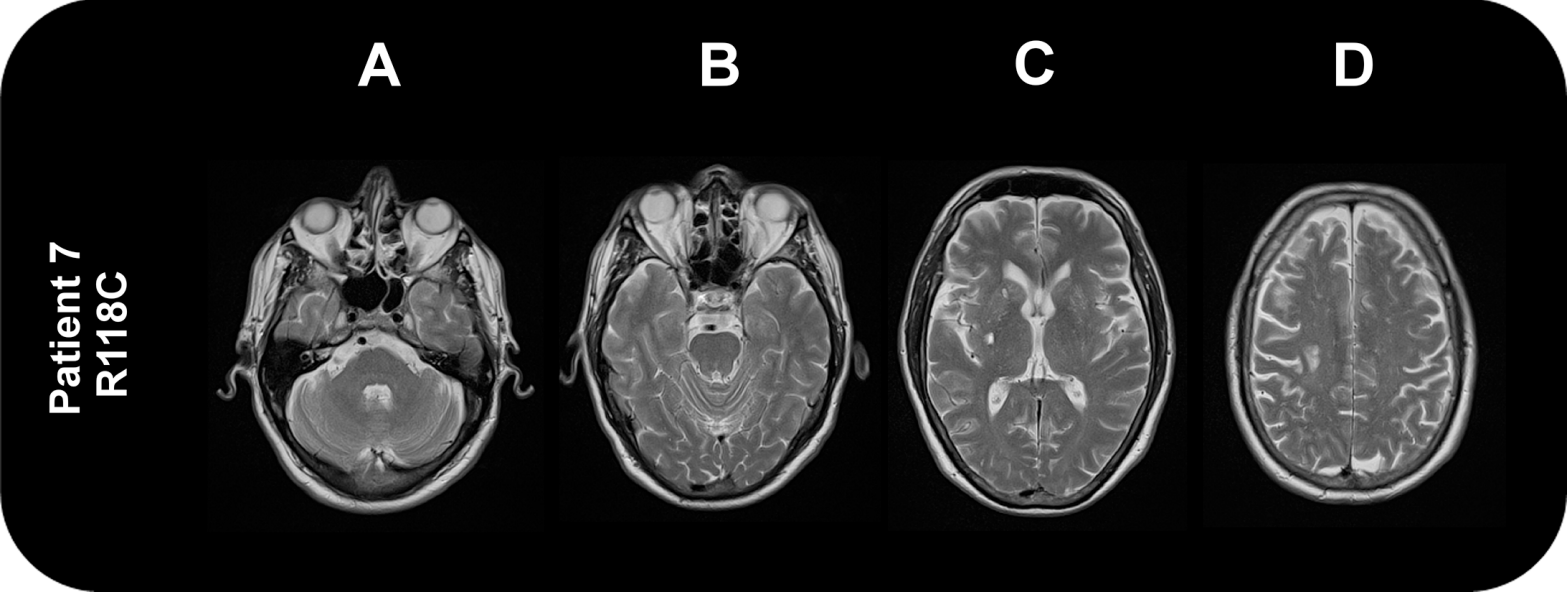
Brain MRI (T2-Weighted) of the patient carrying the *GLA* mutation R118C. Two lacunes are present in the right caudate nucleus and external capsule (C). An area of white matter hyperintensity is present in the peripheral right posterior frontal white matter (D). There were no abnormalities in the posterior circulation.
